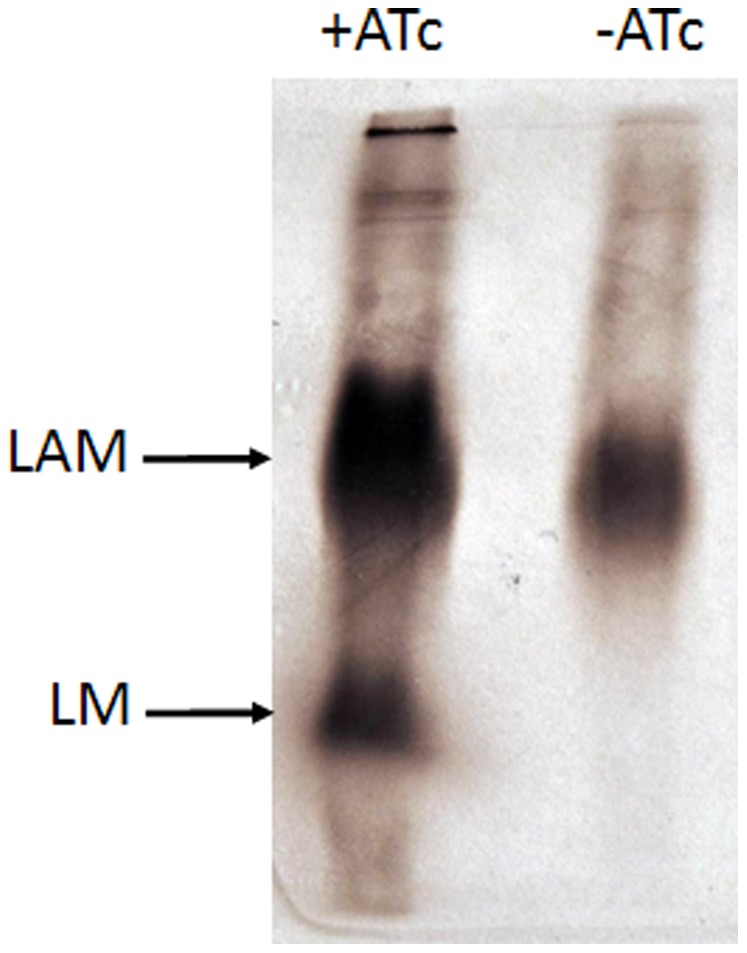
Lipoglycan profile of the ΔMsPpm conditional mutant. [^14^C]-labelled lipoglycan fractions were separated on 15% SDS-PAGE gel from cultures of the ΔMsPpm conditional mutant with or without anhydrotetracycline (ATc). Equal counts (50 000 cpm) were loaded on a 15% SDS-PAGE. Lipoglycans were visualized by 48 h exposure on x-ray films by autoradiography (Kodak Biomax MR film).
